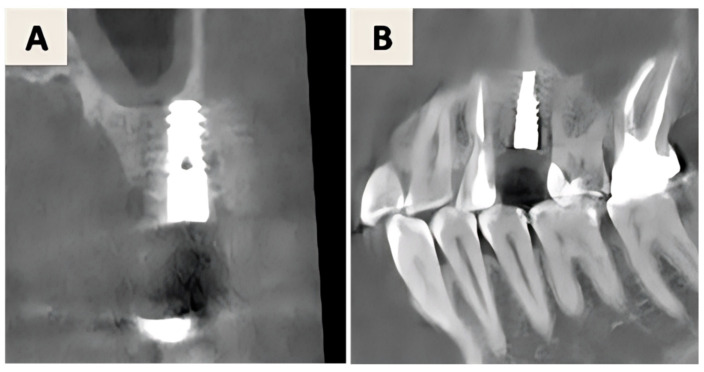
CBCT images in coronal (A) and sagittal (B) plane after 6 months of healing.
